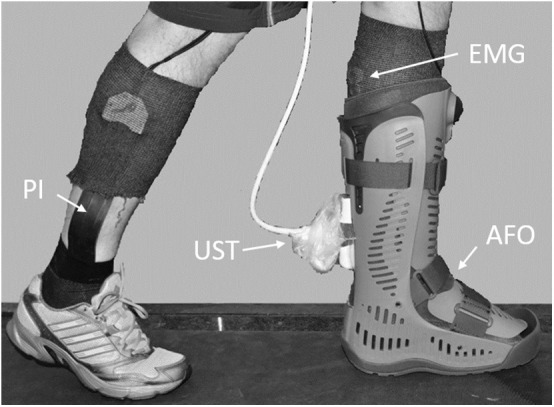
Experimental set up. EMG, emg electrodes; UST, ultrasound transducer; PI, pressure insole; AFO, rigid ankle foot orthosis.
